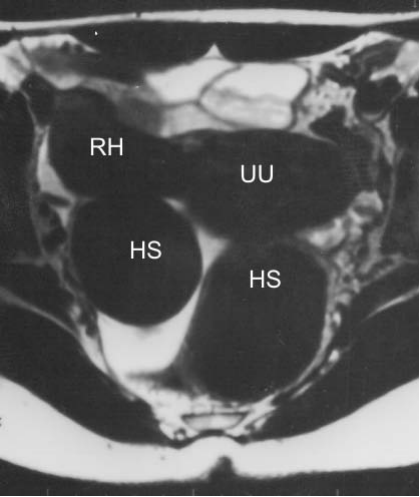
Magnetic resonance imaging showing the unicornuate uterus (UU), the rudimentary horn (RH), and the looped hematosalpinx (HS). T1-weighted MRI of pelvis acquired six days before surgery. The MRI shows the uterus (UU) with a rudimentary horn (RH) in the upper side of the image. The dilated and looped Fallopian tube (HS) can be seen posteriorly to the UU and RH.
